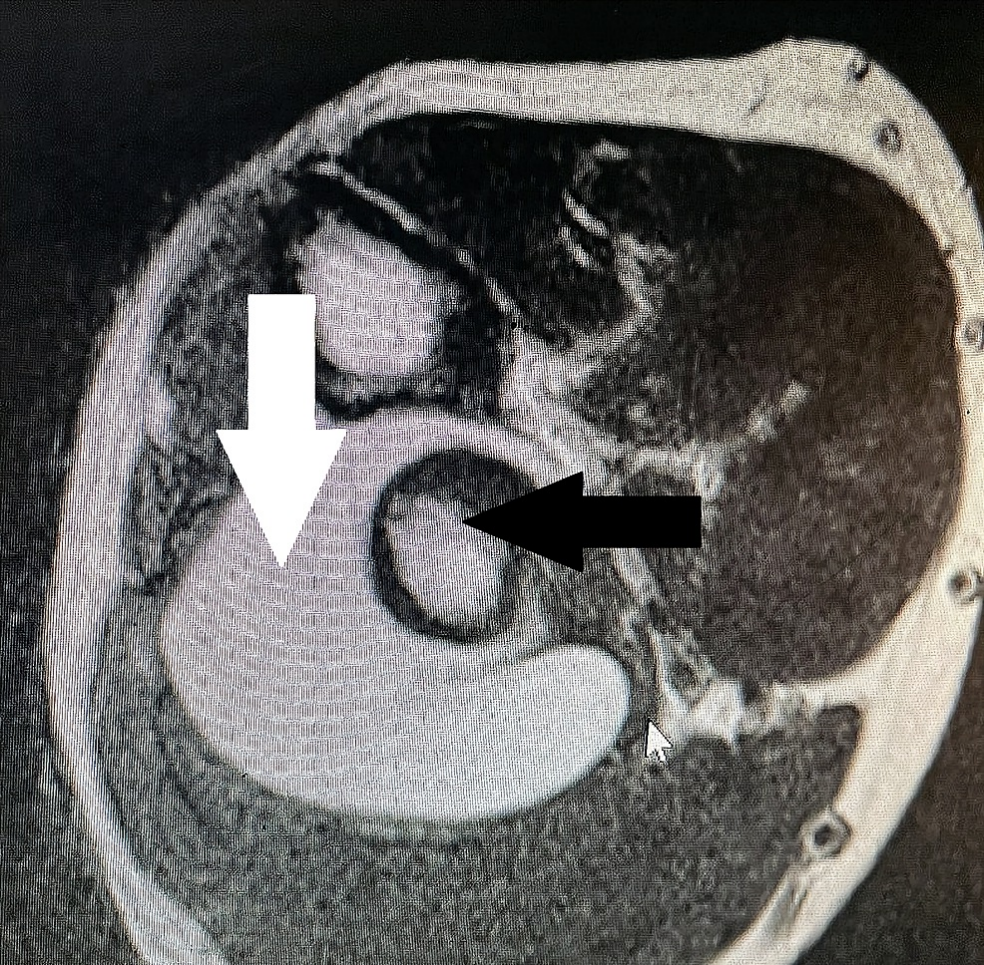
MRI (axial section) of the right forearm showing the circumferential tumor (white arrow) around proximal radius (black arrow) MRI: magnetic resonance imaging
